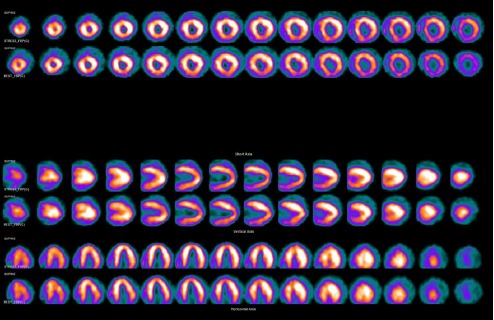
^99m^ Tc-MIBI SPECT images reconstructed with altered patient orientation during processing results in the conventional orientation of cardiac images
